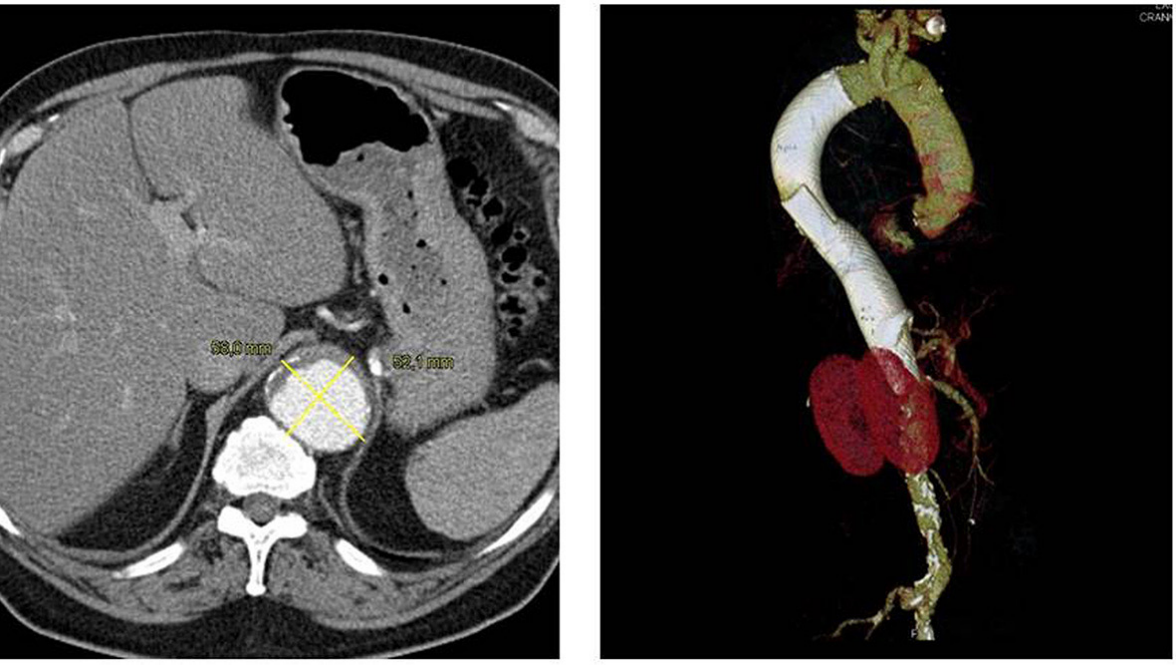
71 year old patient with a thoraco abdominal aortic aneurysm Crawford II classification. Patient was asymptomatic and on Coumadin Therapy for atrial fibrillation. The control CT scans after 1 year show a stable aneurysm diameter although because of the anticoagulation therapy there was no thrombus formation. All visceral branches and both renal arteries were covered by 2 MFM Multilayer stents each 150 cm in length. All branches remained patent during follow up. The patient is still on dual antiplatelet therapy.
